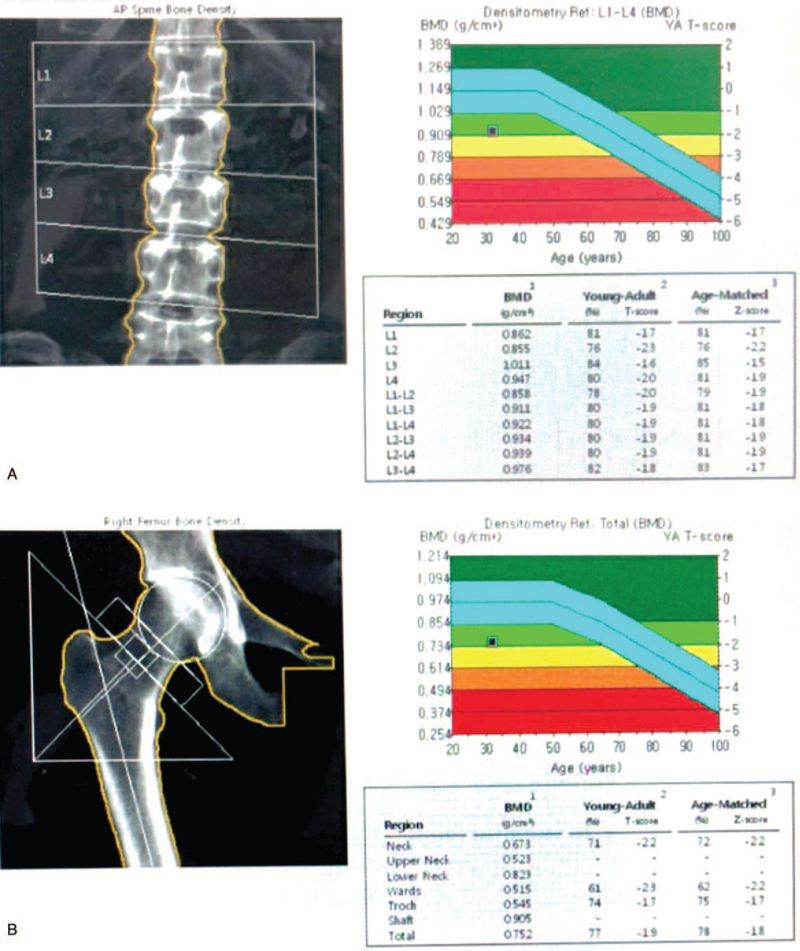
Bone mineral density measured for lumbar spine (A) and femur (B) by dual-energy x-ray absorptiometry after long-term follow-up.
